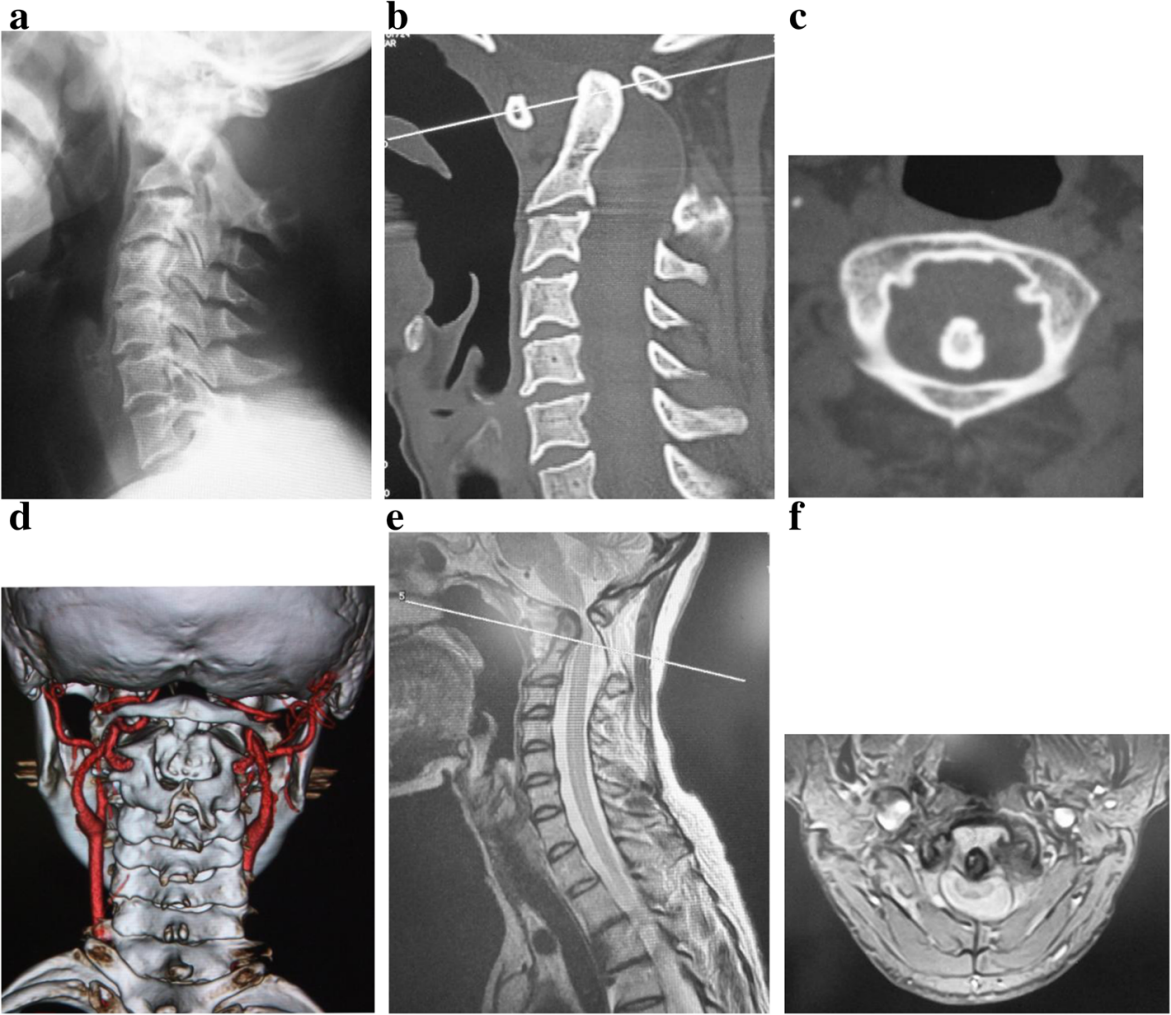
Preoperative imaging. a The cervical neural position showing expansion of the atlanto-dental interval. The upper atlantodental interval was 11.8 mm. b Computed tomography showing enlargement of the C2/3 foramen on the sagittal side of the left cervical spine. c Axial computed tomography image at the height of the C2 vertebra showing enlargement of the left side C2/3 foramen. d Computed tomographic angiography showing a left-side vertebral artery inside of the C1 lamina. e T2-weighted magnetic resonance imaging of the sagittal cervical spine showing spinal cord compression caused by a dislocated odontoid. f T2-weighted magnetic resonance cervical axial imaging showing disruption of the transverse ligament of the atlas and neurofibroma between the anterior arch of C1 and the odontoid
